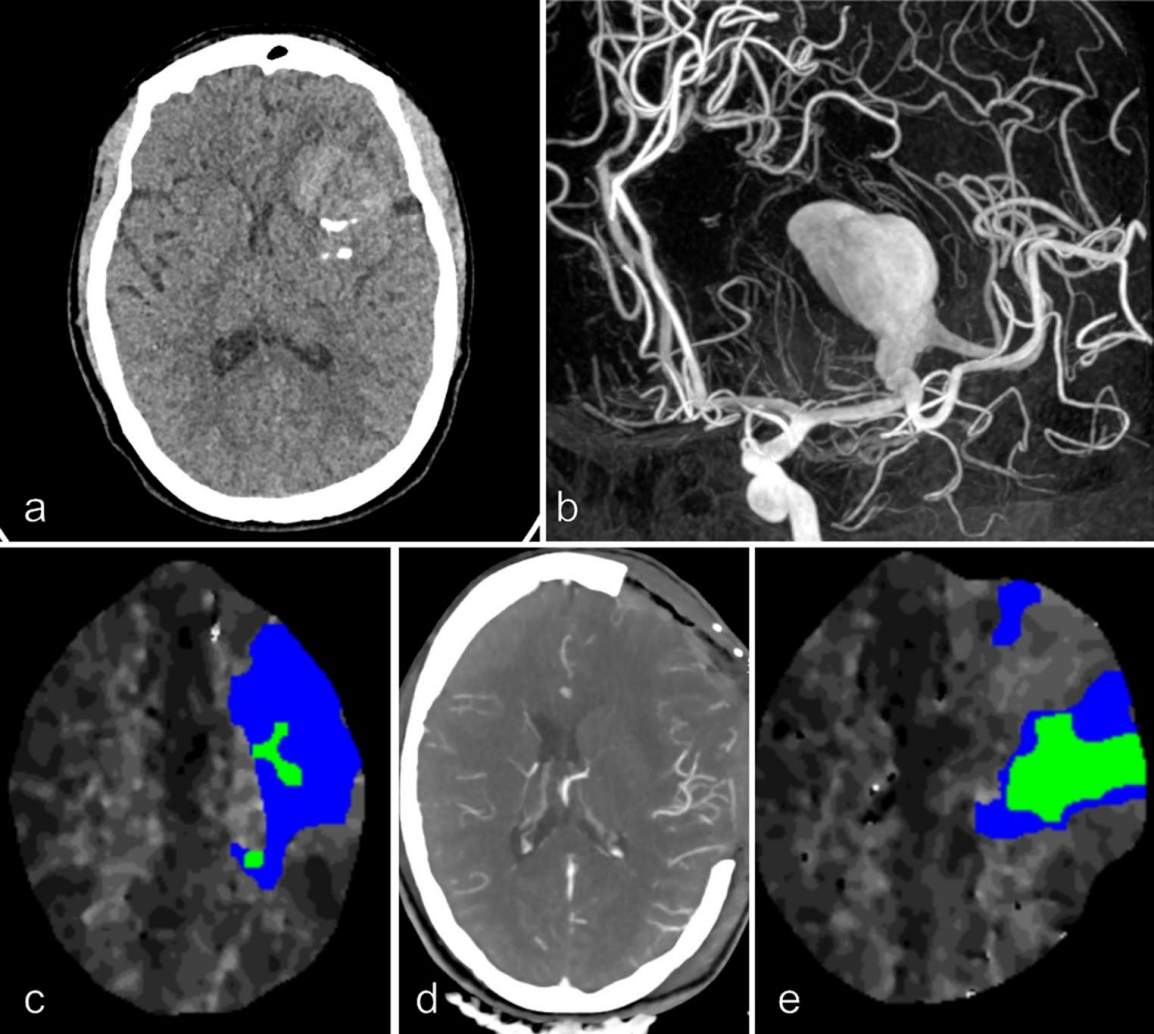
Patient 9, 44 yo female, without any medical history, complained about increasing headache for 3 weeks. CT scan, MRI/MRA and conventional angiography demonstrated a left giant MCA aneurysm (maximal diameter = 50 mm). A moderate peri-aneurysmal edema and intrasaccular thrombus were noted. The midline brain shift was 5 mm (a and b). A severe hypoperfusion in the left distal MCA territory was noted on the perfusion CT scan (c). A distal STA-MCA bypass was performed, followed by aneurysmal trapping and partial resection of the aneurysmal sac. A deliberate decompressive hemicraniectomy was performed at the end of the procedure. The patient was extubated after 24 h without any neurological deficit (d). An improvement of the hypoperfusion was recorded on control Perfusion CT scan (e). A long-term favorable outcome was recoded (mRS score = 1)
